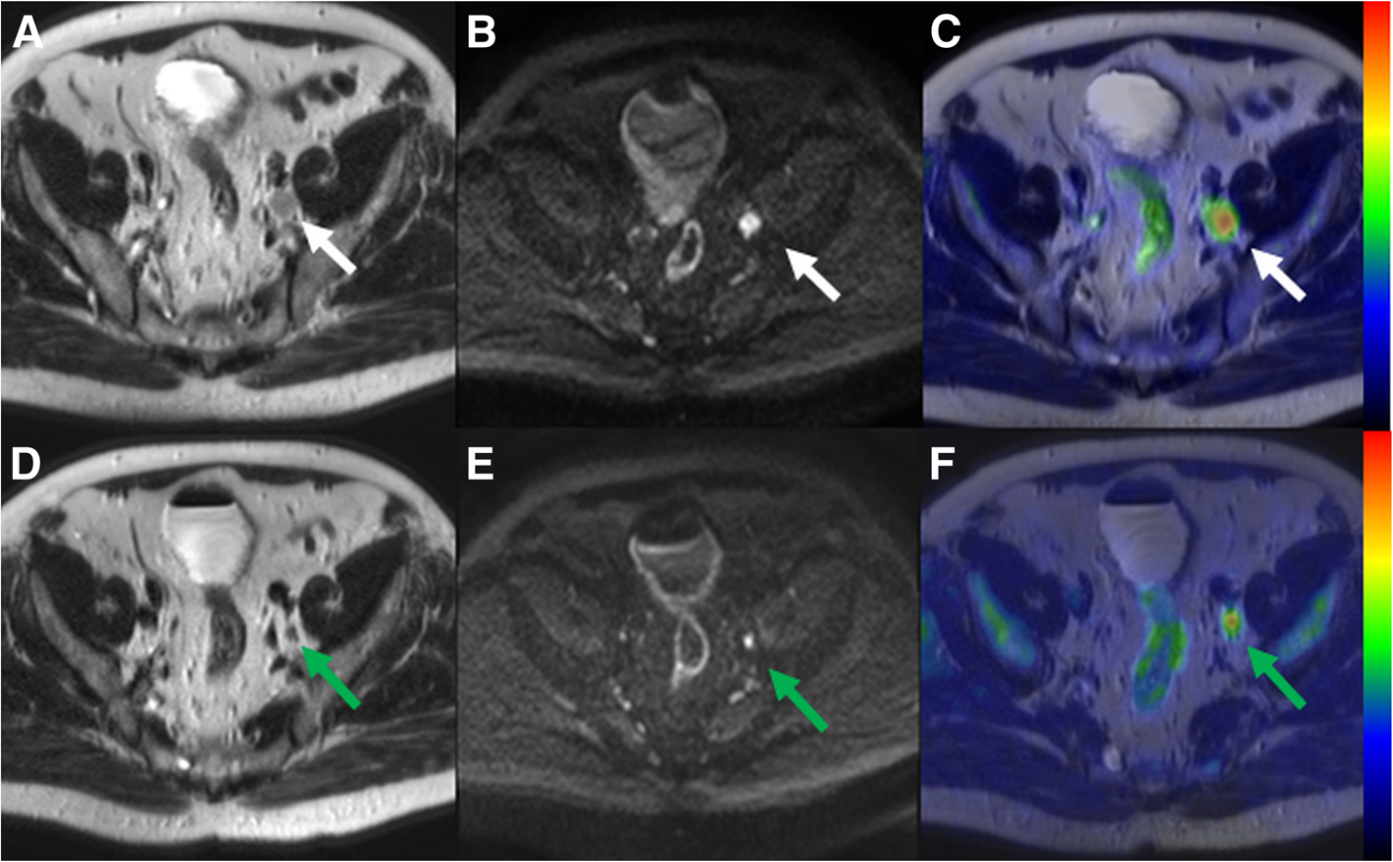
Comparison of lymph node staging (N-stage) on pre-transurethral resection (a, b, c) and post-chemotherapy ^11^C-acetate PET/MRI (d, e, f) in patient number 12, the same patient as in Fig. 4. 17 mm right obturator lymph node (a - white arrow on T2-weighted image) demonstrated increased diffusion signal restriction (b - b value 800 s/mm^2^ trace diffusion weighted image) and increased ^11^C-acetate uptake (c - PET fused with T2-weighted image, SUV is scaled from 0.0 to 3.5) suggestive of lymph node metastasis. On post-chemotherapy ^11^C-acetate PET/MRI, lymph node decreased in size and measured 4 mm (d - green arrow on T2-weighted image) with increased diffusion signal (e - b value 800 s/mm^2^ trace diffusion weighted image) and increased ^11^C-acetate uptake (f - PET fused with T2-weighted image, SUV is scaled from 0.0 to 3.0). SUVmax values of the lymph node on the pre-transurethral resection (c) and post-chemotherapy ^11^C-acetate PET/MRI (f) were 3.4 and 2.8, respectively. The lymph node was confirmed to be lymph node metastasis measuring 3 mm on extended pelvic lymph node dissection, thus the findings of ^11^C-acetate PET/MRI were considered as true positive
